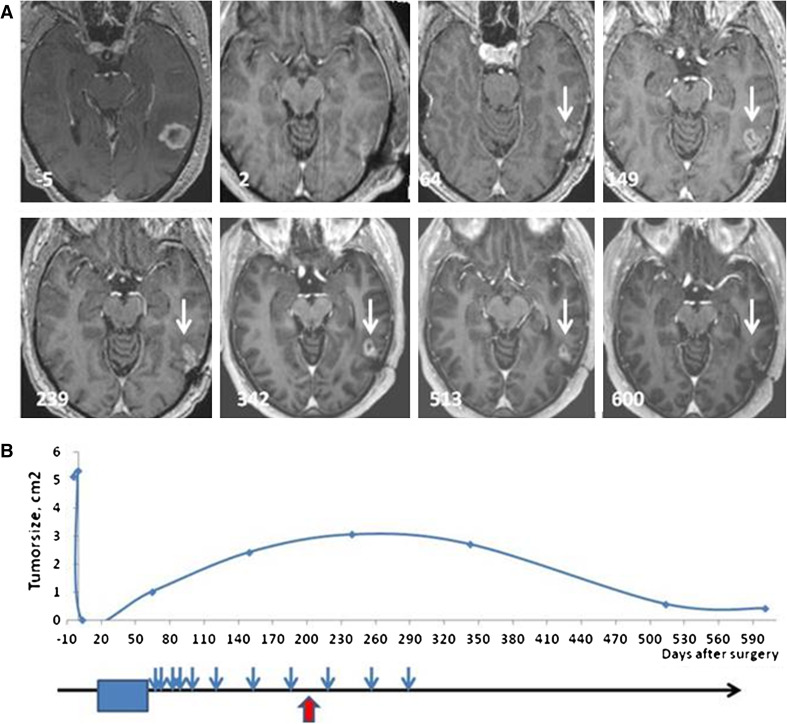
Changes in size of contrast-enhancing tumor over time. a Brain MRI axial T1 images after intravenous gadolinium contrast in patient #5. Days before (negative) and after surgery are noted on the MRI scans. No residual tumor was observed post-operatively (day 2), but at the end of the 6 weeks course of combined chemo/radiotherapy, a small contrast-enhancing lesion could be detected at the anterior margin of the resection cavity, as indicated with the *white arrow* (day 64). b Maximal area of contrast enhancement plotted against days since surgery (abscissa). *Lower part* of the figure indicates the timing of concomitant chemo-radiotherapy (*blue box*), DC vaccinations (*blue arrows*), and immune response (*red arrow*)
